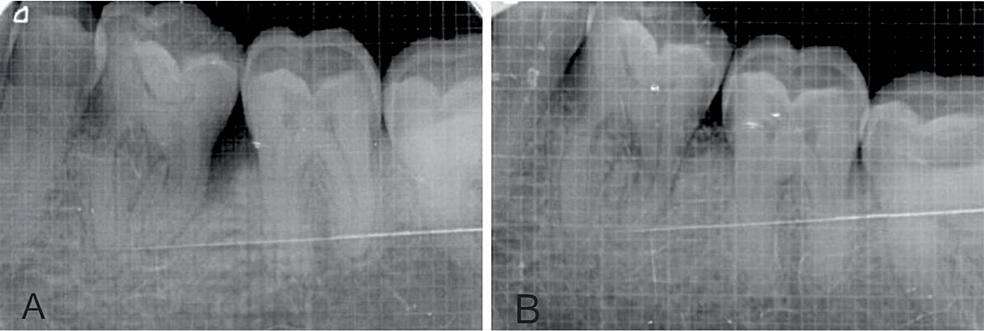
(A) Radiograph showing the bone level at baseline at site one (BCP). (B) Radiograph showed the bone level at six months at site one (BCP). BCP: biphasic calcium phosphate.
